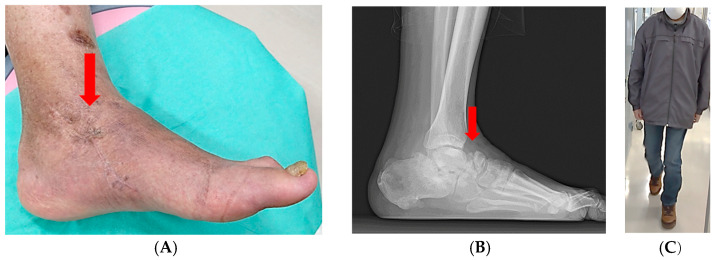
Long-term outcome at 4-year follow-up. (A) Clinical appearance showing the medial ankle without signs of active inflammation (red arrow). (B) Lateral radiograph demonstrating residual Charcot-type destructive changes involving the talonavicular and subtalar joints without evidence of recurrent infection (red arrow). (C) Clinical outcome demonstrating independent ambulation without reliance on orthotic support. Limb salvage was maintained with no recurrence of infection at 4 years, representing sustained infection-free survival in a severely compromised host.
